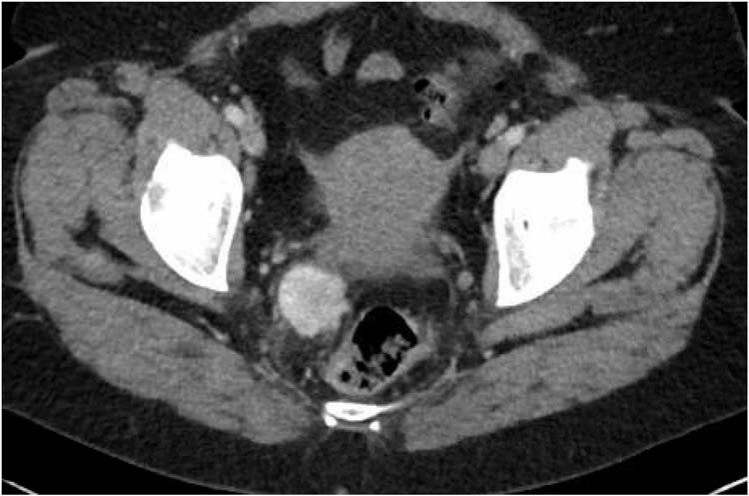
Latest CT evaluation prior to consultation, showing a right pararectal hypervascular mass.
